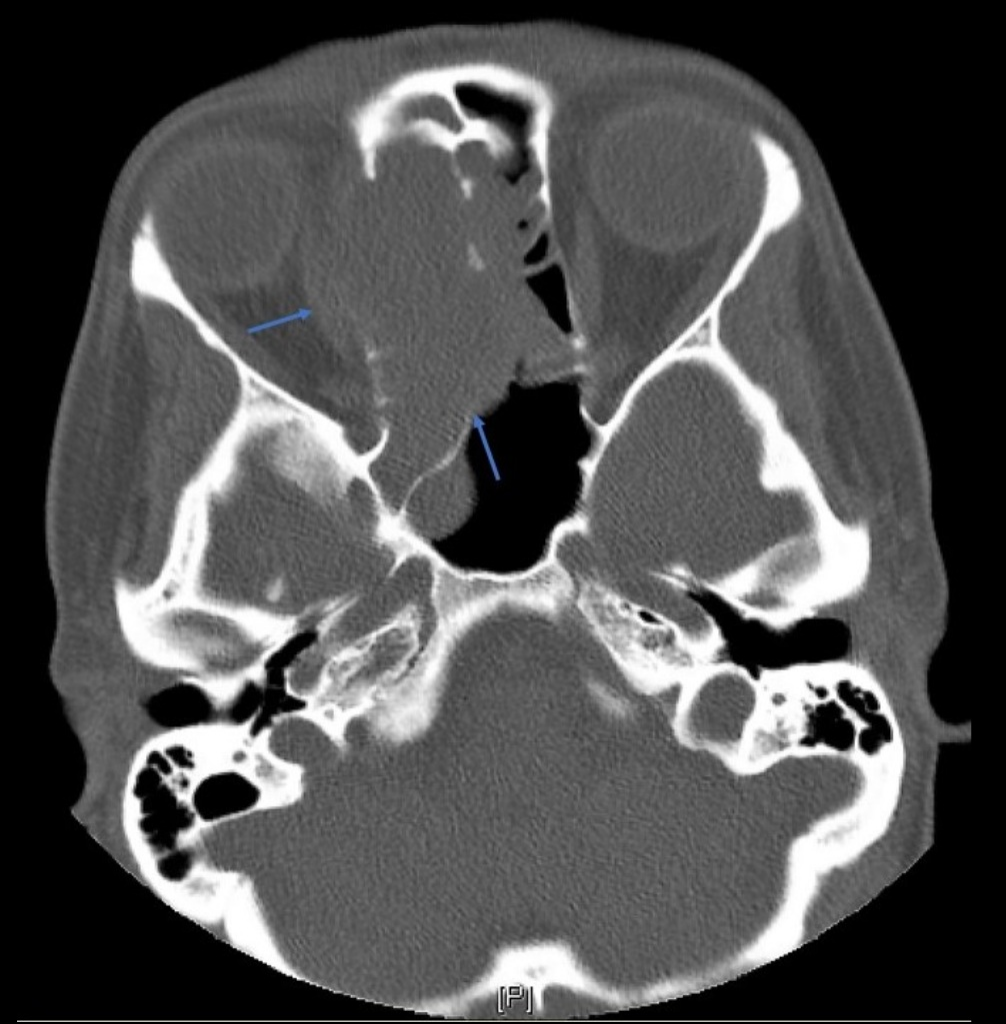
Transverse computed tomography (CT) image demonstrates a 2.6 x 4 x 4.3 cm mass
